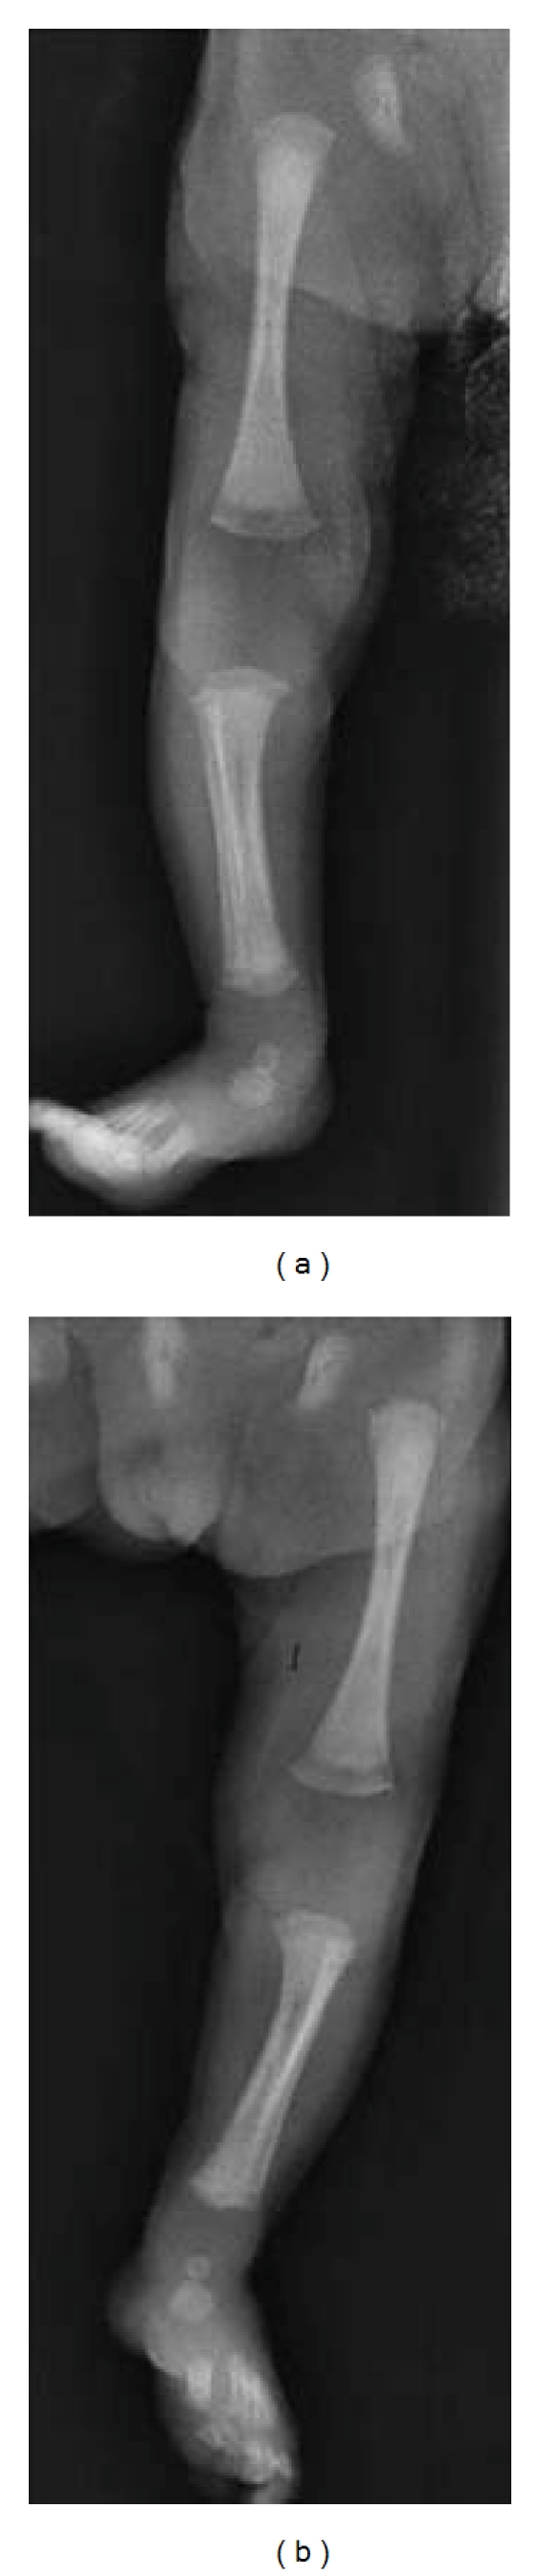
X-ray examination of the long bones of the case described.
